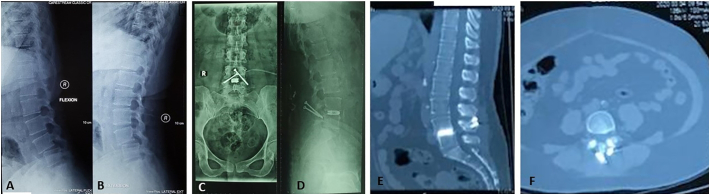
A 58-year-old female with low back pain for more than one year. Radiological examination reveals spondylolisthesis on level L4-L5 (A, B). The patient underwent the translaminar screw fixation and showed no sign of pain. X-ray and CT Scan show union on level L4-L5 (C–F).
